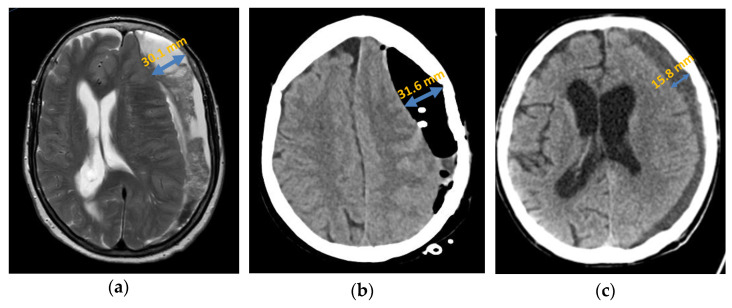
Axial presentation chronic subdural haematoma: (a) preoperative septation in axial plane in MRI; (b) postoperative subdural air accumulation in CT; (c) recurrence of chronic subdural haematoma in CT.
